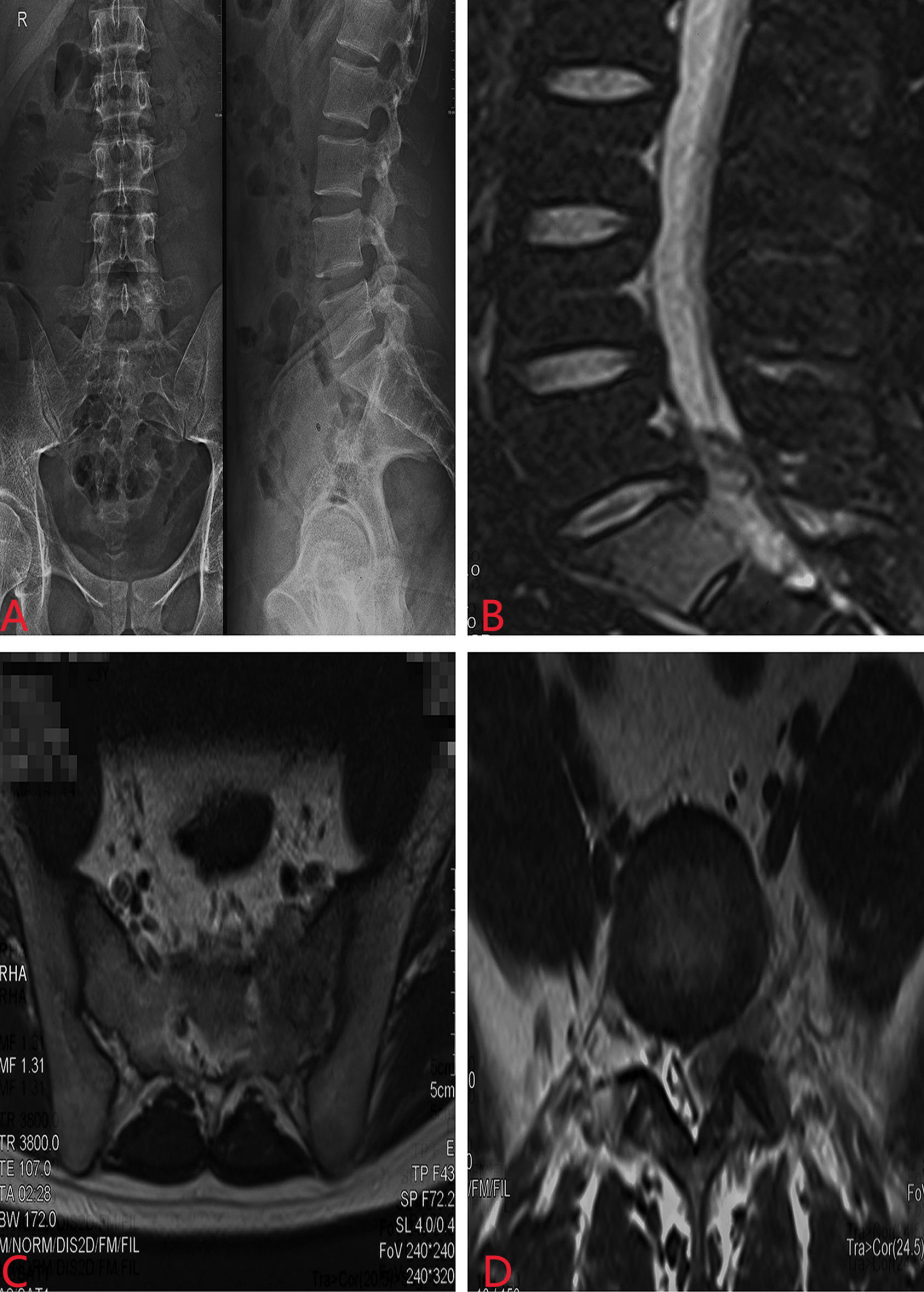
Preoperative X-ray and MRI revealed a space-occupying lesion with abnormal signal of the sacrum at the S1 level. (A) X-ray of the lumbosacral spine; (B) sagittal MRI; (C) coronal MRI; (D) lateral MRI.
